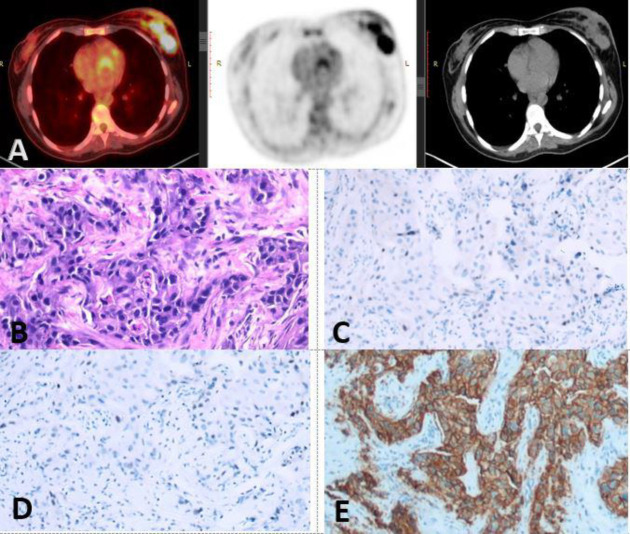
[^18^F]-FDG PET/CT fused, CT and PET images revealed: metabolically active left breast ill-defined soft tissue lesion measuring 4.1×2.5 cm with SUV_max_ 8.5, MTV 28.89 cm^3^ and TLG 152.55 SUV-bw×cm^3^ (A). Hx & E slide of a case of invasive duct carcinoma grade III (B), ER: Negative (Allred 0/8) (C), PR Negative, Allred (0/8) (D), HER-2: Positive score 3+, ×200 (E)
